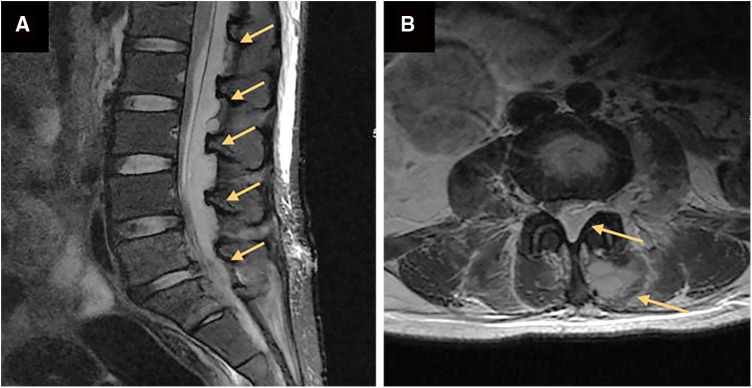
Magnetic resonance images of the lumbar before percutaneous endoscopic drainage in axial (A) and sagittal (B). (A) a long segment epidural abscess from L1 to S1 (orange arrow). (B) Massive epidural abscesses compressing the spinal cord and nerve roots with paravertebral involvement (orange arrow).
